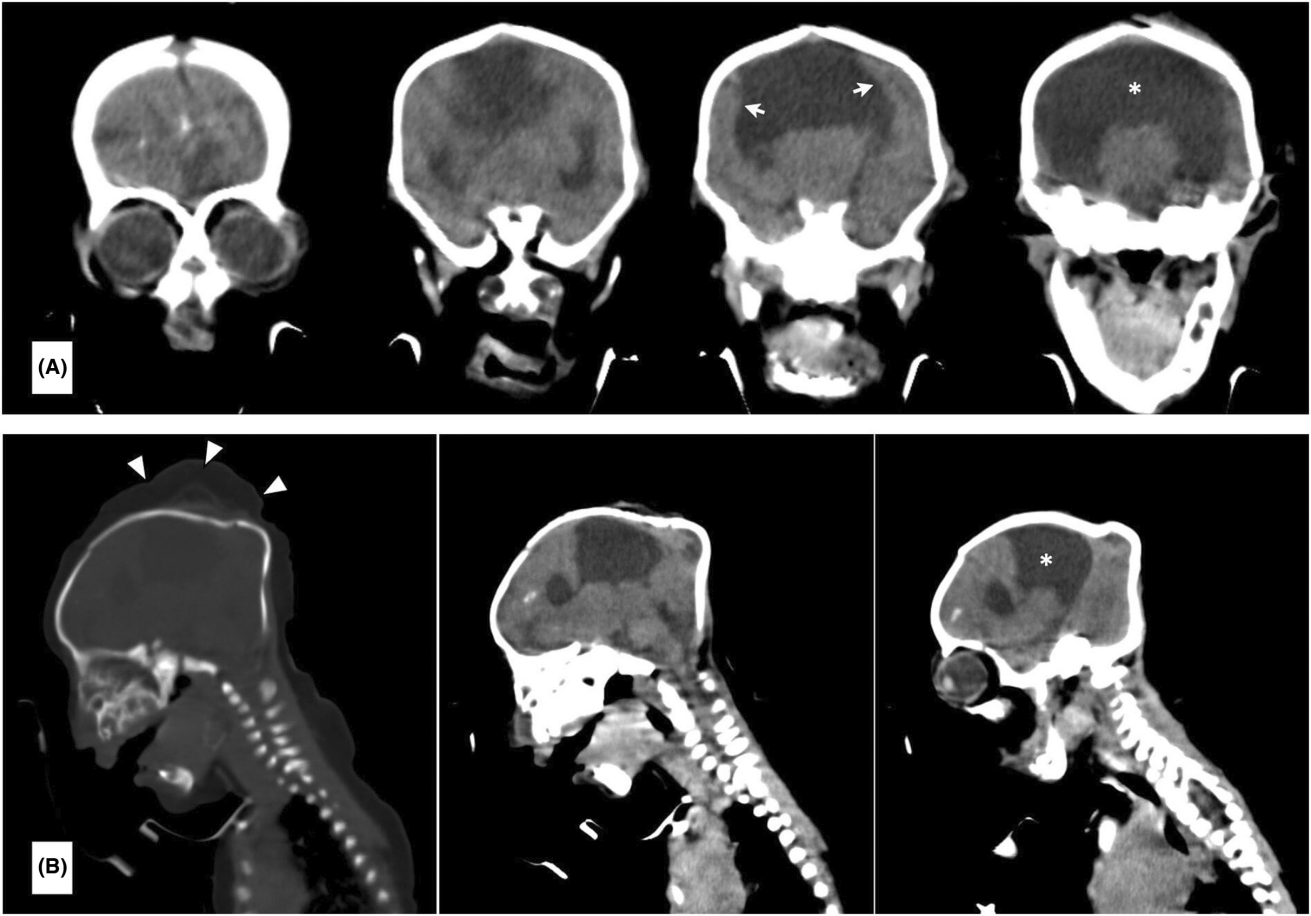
Non‐contrast CT scan of the brain in coronal (A) and sagittal (B) views showing a monoventricle (asterisk), fused cortex (white arrows), and cephalohematoma of the vertex (white arrowheads).
